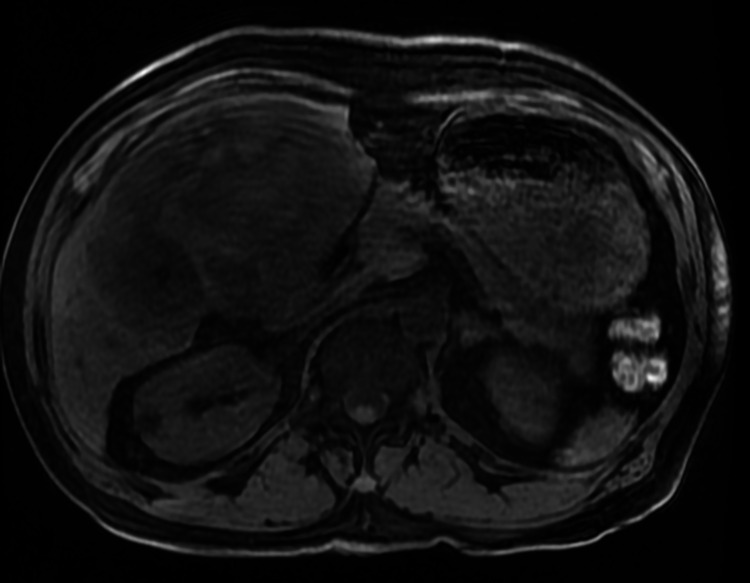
MRI liver (axial view) showing large heterogenous mass involving most of right hepatic lobe with extension into left lobe. MRI: magnetic resonance imaging.
